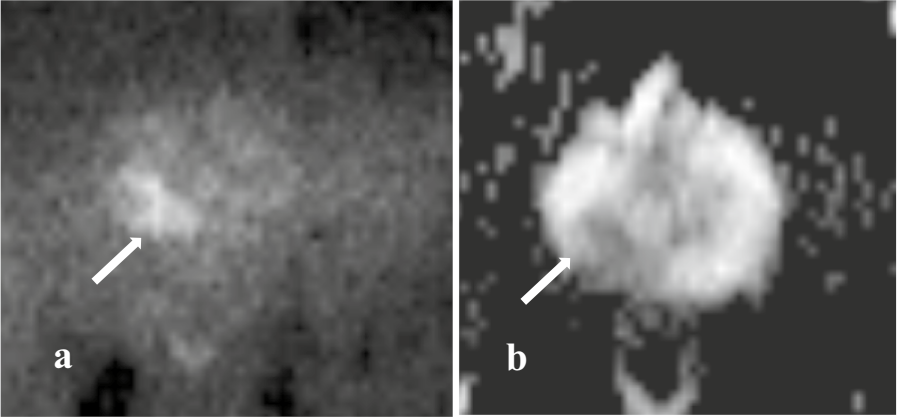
Pelvic MRI revealed a high-intensity area in the right lobe on DWI (a: white arrow) and a low-intensity area for ADC (b: white arrow)
